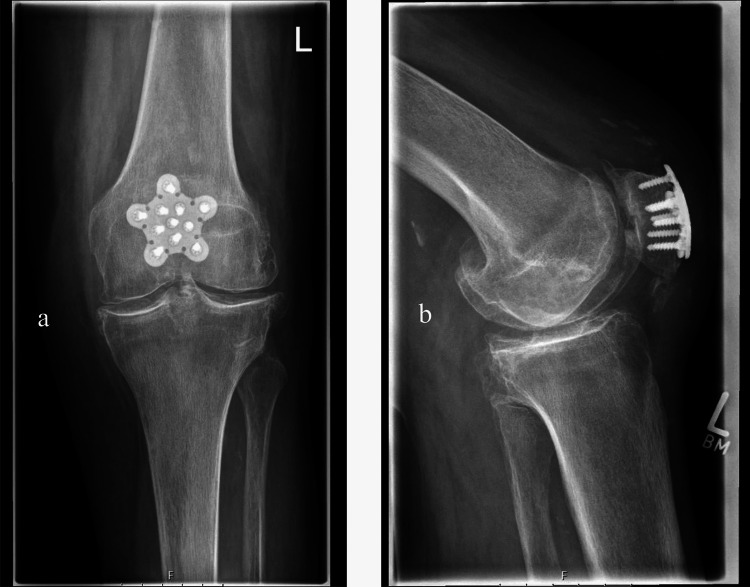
Anteroposterior (a) and lateral (b) X-rays showing a multifragmentary patellar fracture following operative fixation with a locking plate Reproduced from Pesch et al. [28], licensed under Creative Commons Attribution 4.0 International License (CC BY 4.0).
